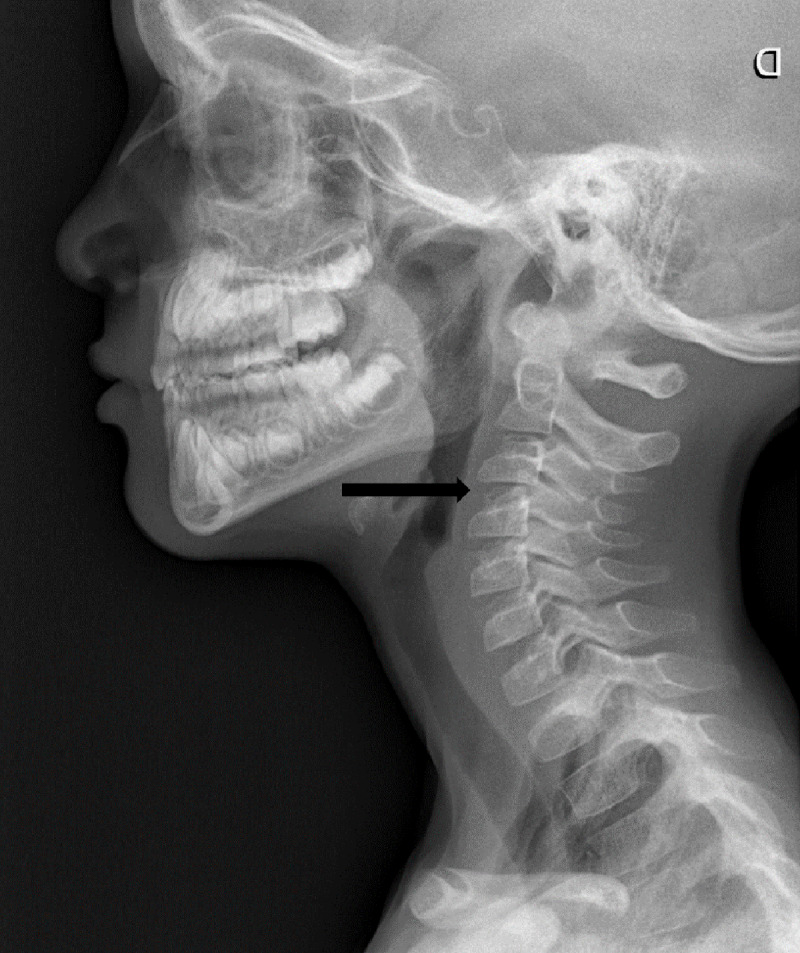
Initial lateral cervical X-ray where a discrete C3-C4 dislocation was described.
